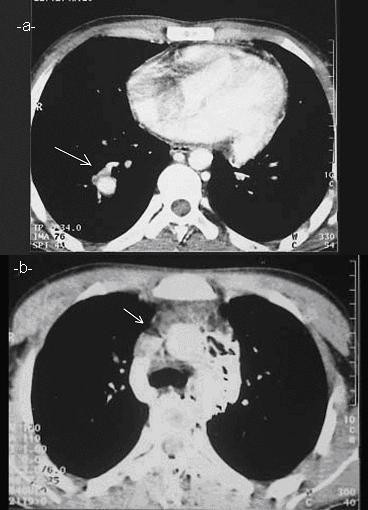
Chest helical computed tomography demonstrating a single (14 mm) right main pulmonary artery aneurysm and image of thrombi in the right heart (a), diffuse venous collateral vessels and superior vena cava thrombosis (b).
